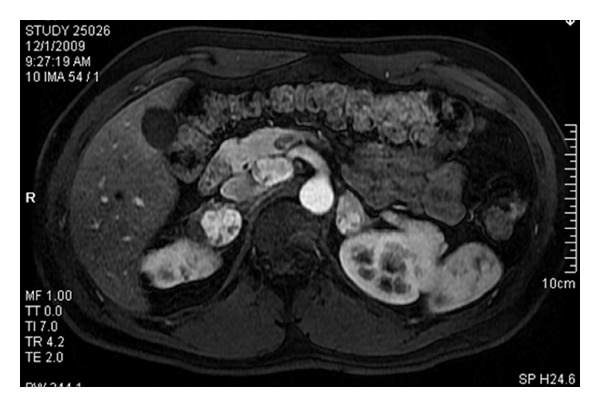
Arterial phase T1-weighted image in the axial plane following intravenous contrast depicts hypervascular adrenal masses on both sides and an approximately 3 cm hypervascular mass in the uncinate process of the pancreas consistent with a neuroendocrine tumor.
